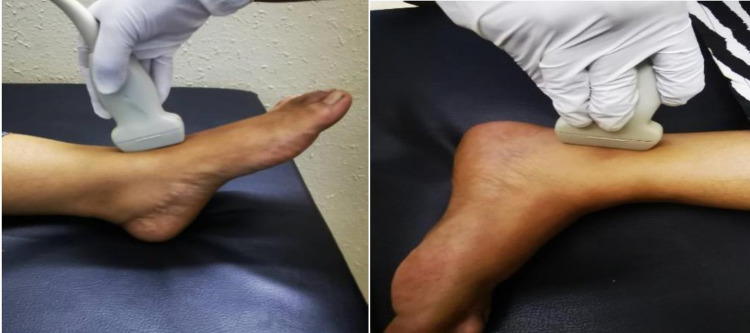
The image shows the sonographic scanning technique for DPA and PTA. The photo was taken from a participant in the study.
